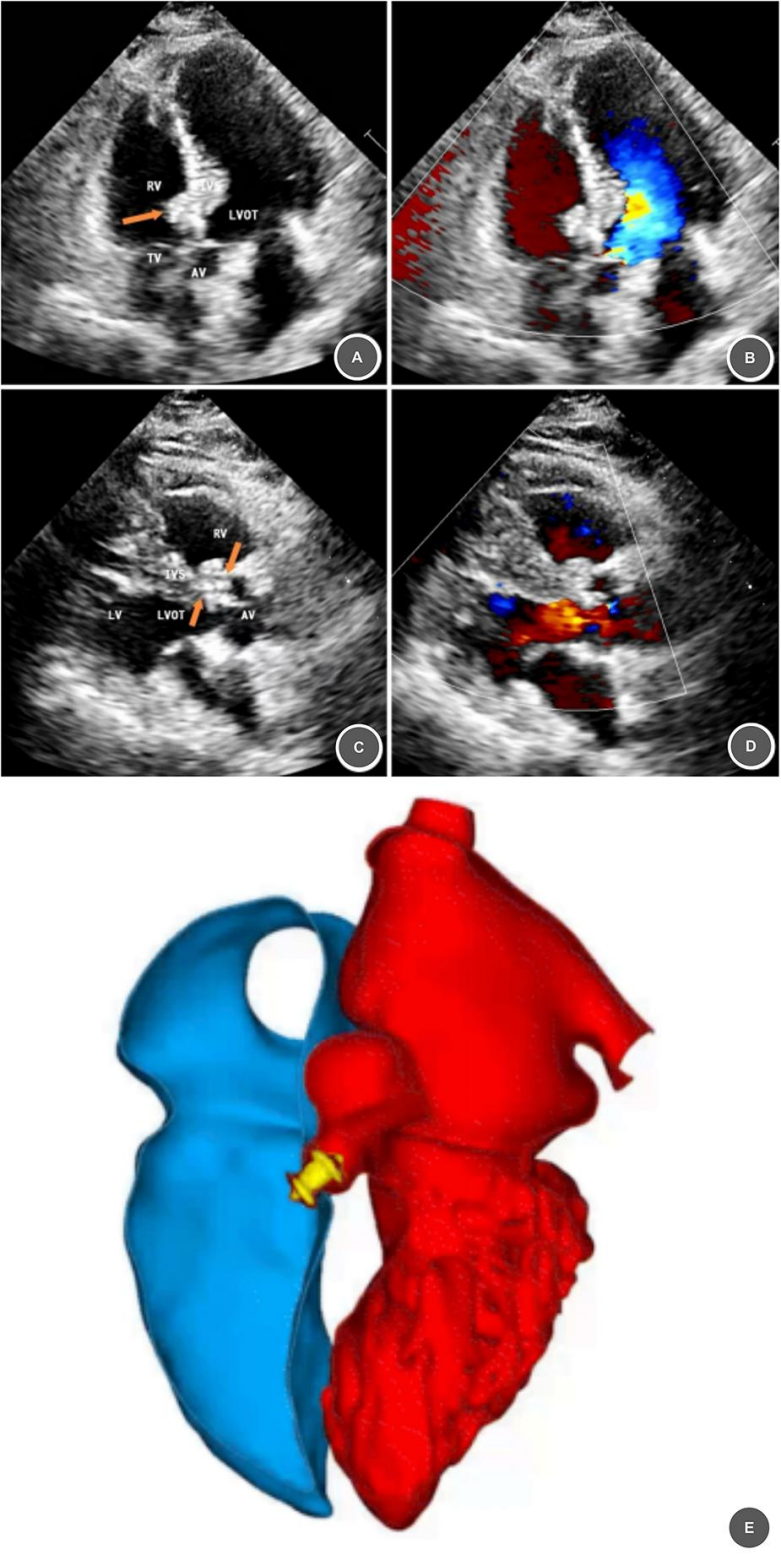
One–month postoperative follow, up imaging after VSD closure. (A–D) One-month postoperative echocardiography demonstrating a well-positioned and stable occluder with no significant residual shunt (arrow). (E) One-month postoperative cardiac CT (cardiac cavity reconstruction and rendering) showing localized metallic density in the ventricular septum, confirming stable device morphology and position.
